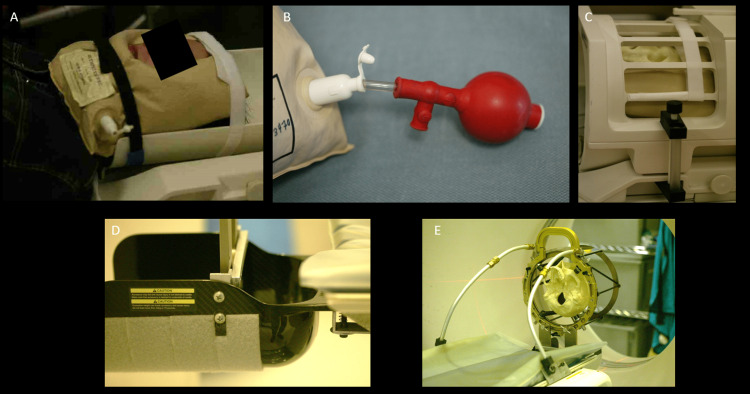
Methods of motion stabilization during MRI and CT imaging. In the absence of rigid skull fixation such as for a preoperative MRI, an MRI-compatible bean bag can be used to stabilize the head (A). A suction tool can be used to remove air from the bean bag which is affixed to the patient (B). This then can fit within the parameters of the MRI coil (C). When the head is fixated in a stereotactic apparatus, such as for a CT scan sequence for stereotactic localization, a posterior bracket attached to the stereotactic frame can be used to support the head (D). If further fixation is needed, rod brackets can be used to support the stereotactic frame anteriorly (E). These rod brackets (E) are not routinely used in our center, but the posterior bracket (D) is used for all cases, such as for the good and bad image sequences. Head-frame motion can still occur during imaging acquisition despite these methods to reduce motion and careful analysis is needed for all the final images in each sequence. MRI - Magnetic Resonance Imaging, CT - Computed Tomography, Images courtesy of Eric Sabelman, PhD and Gary Heit, PhD, MD
